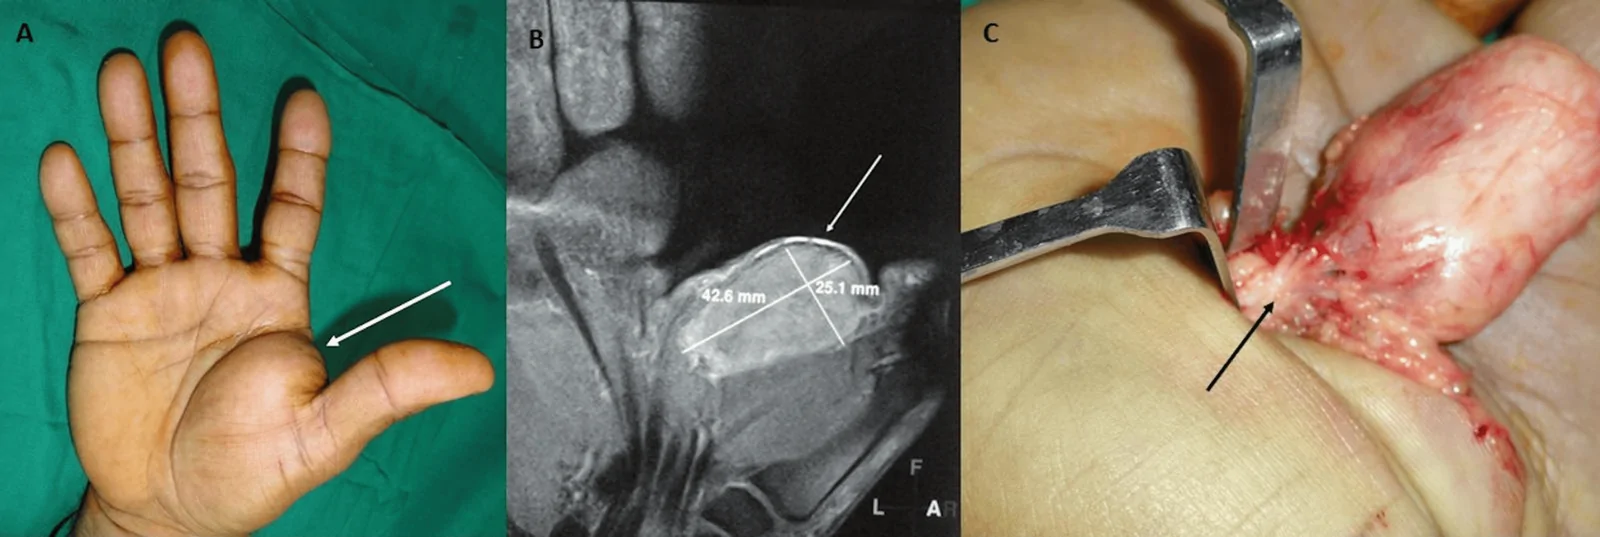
Clinical, radiological, and intraoperative findings of a right thenar swelling (A) Swelling in the thenar region extending into the first web space. (B) MRI demonstrating a well-capsulated, lobulated lesion measuring 42.6 × 25.1 mm with incomplete fat suppression. (C) Intraoperative view showing origin of tumor from a muscular branch of the median nerve MRI: magnetic resonance imaging
